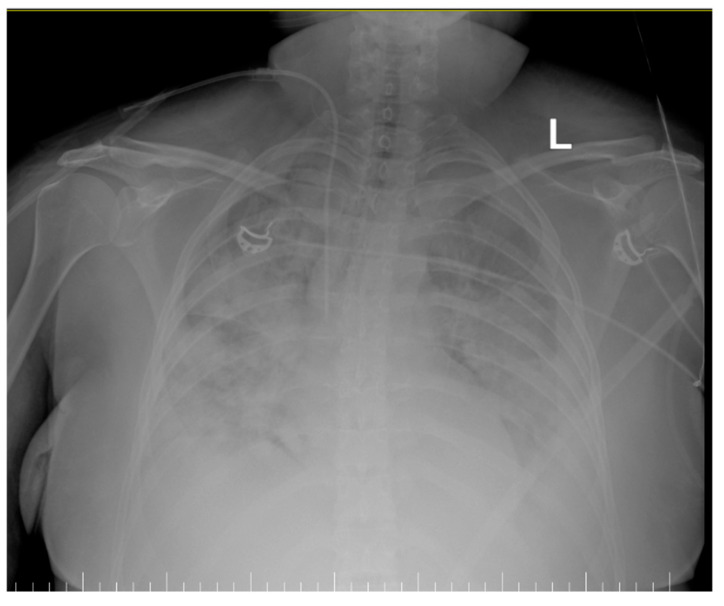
Thoracic X-Ray showing diffused bilateral infiltrates (L-left).
